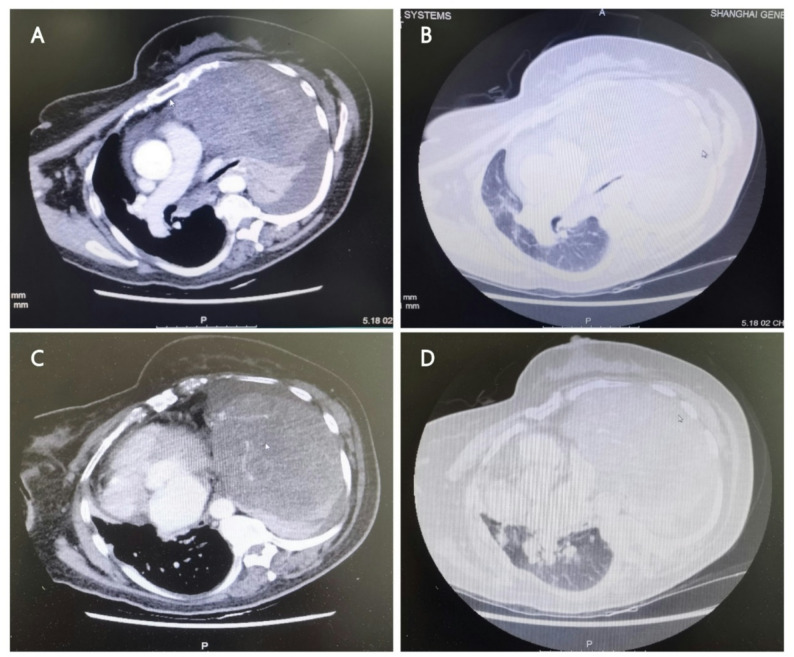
Chest CT scan preoperative. Left giant mediastinal tumor
